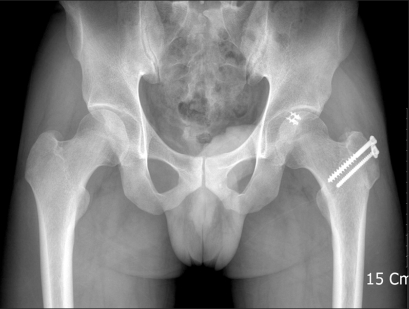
Postoperative radiograph shows union of the osteotomy site and no arthritic changes.
